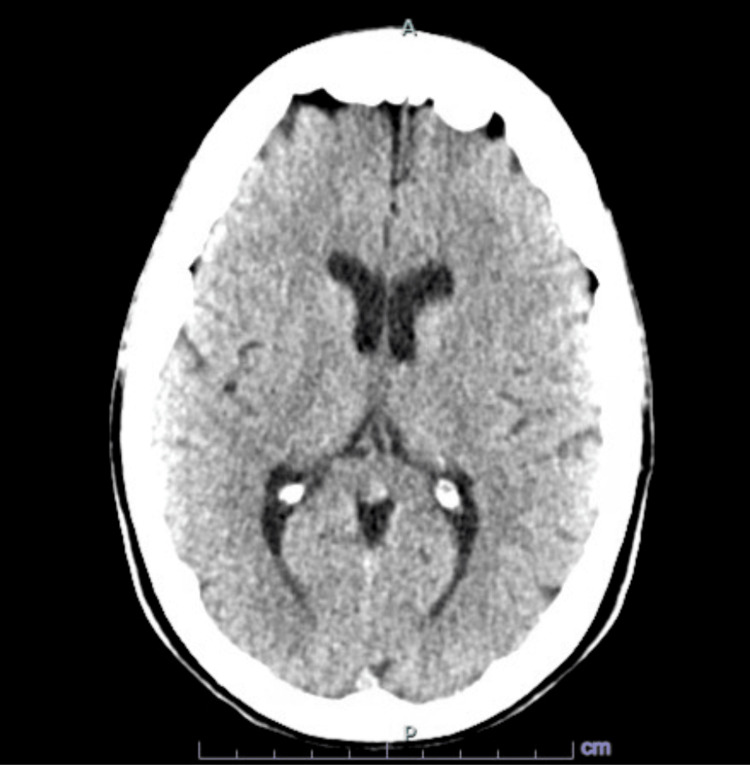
Axial non-contrast CT of head No acute intracranial hemorrhage, mass effect, or midline shift noted. Gray-white matter differentiation is preserved.
